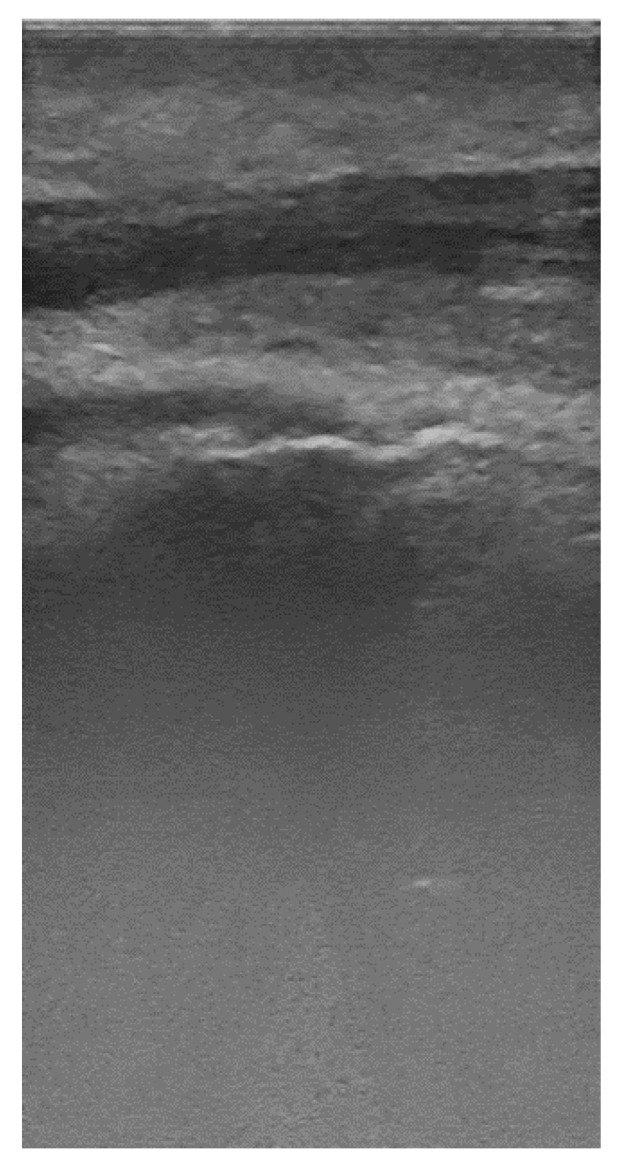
Upper premolar, periodontal ultrasound image, 20 MHz.
